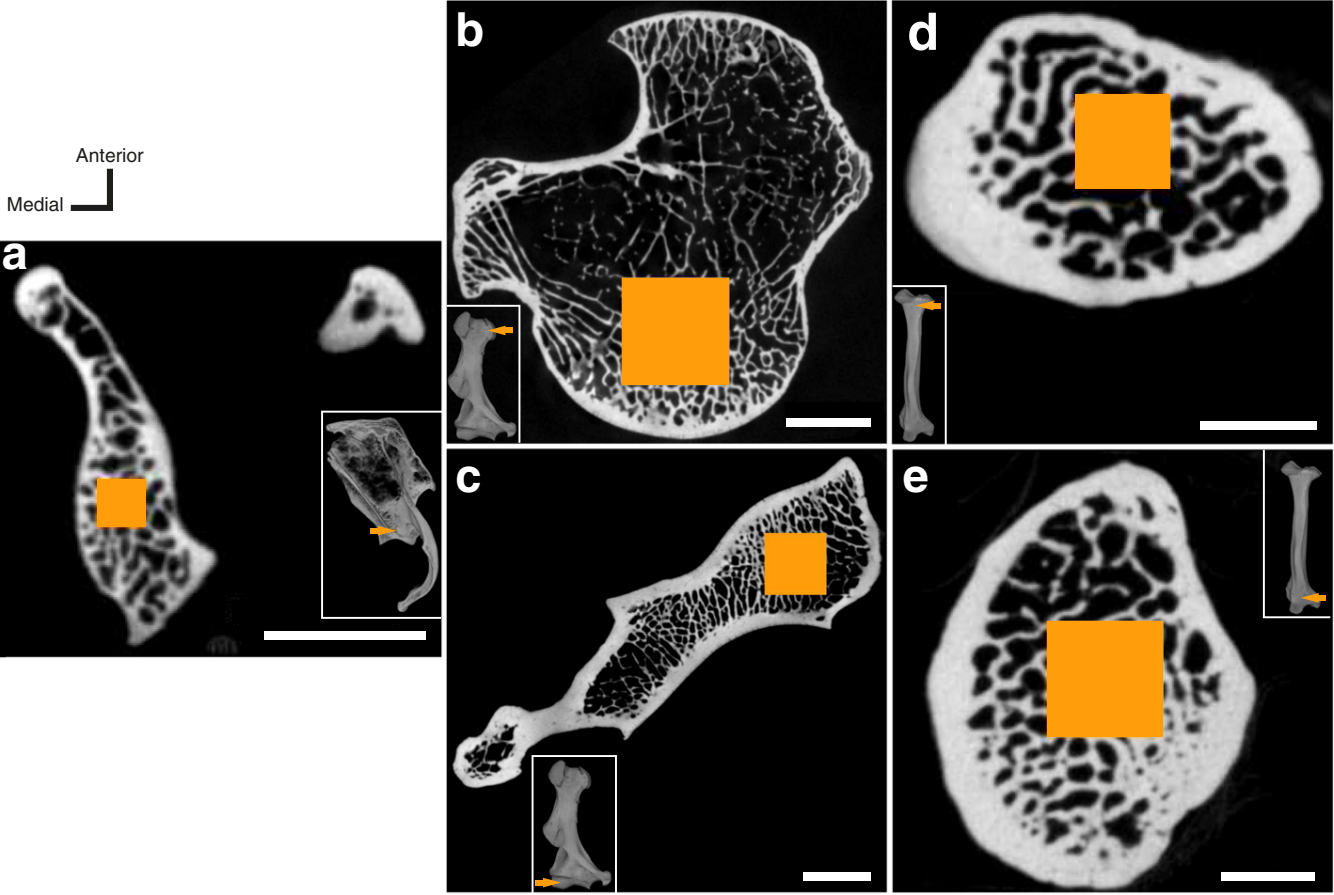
Selection of the regions of interest (ROIs). Transverse virtual sections (CT-scans in the X-Y plane) of the studied bones oriented as for the data acquisition, where the area coloured in orange indicates the central (transverse) slice in the ROI. a glenoid fossa (scapula of *Chlamyphorus truncatus* ZMB_Mam_6007), scale bar = 3 mm; (b) and (c) humeral head and capitulum, respectively (*Cabassous tatouay* SMNS-26661), scale bars = 5 mm; (d) and (e) radial head and trochlea, respectively (*Euphractus sexcinctus* SMNS-26660), scale bars = 2 mm. Three-dimensional (3D) renderings of the relevant bones (right bones seen in lateral view for the scapula and anterior view for the humerus and radius) are displayed as insets. The anatomical orientations (‘Anterior’, ‘Medial’) are only valid for the sections (not for the 3D renderings). ROIs were selected to be as large as possible but excluding the cortex. Note that the slices displayed (centre of each ROI) do not appear as comprising the maximal quantity of trabeculae because the ROI selection was always restricted at its proximal or distal end. See also 3D models in Additional files 2, 3, 4
